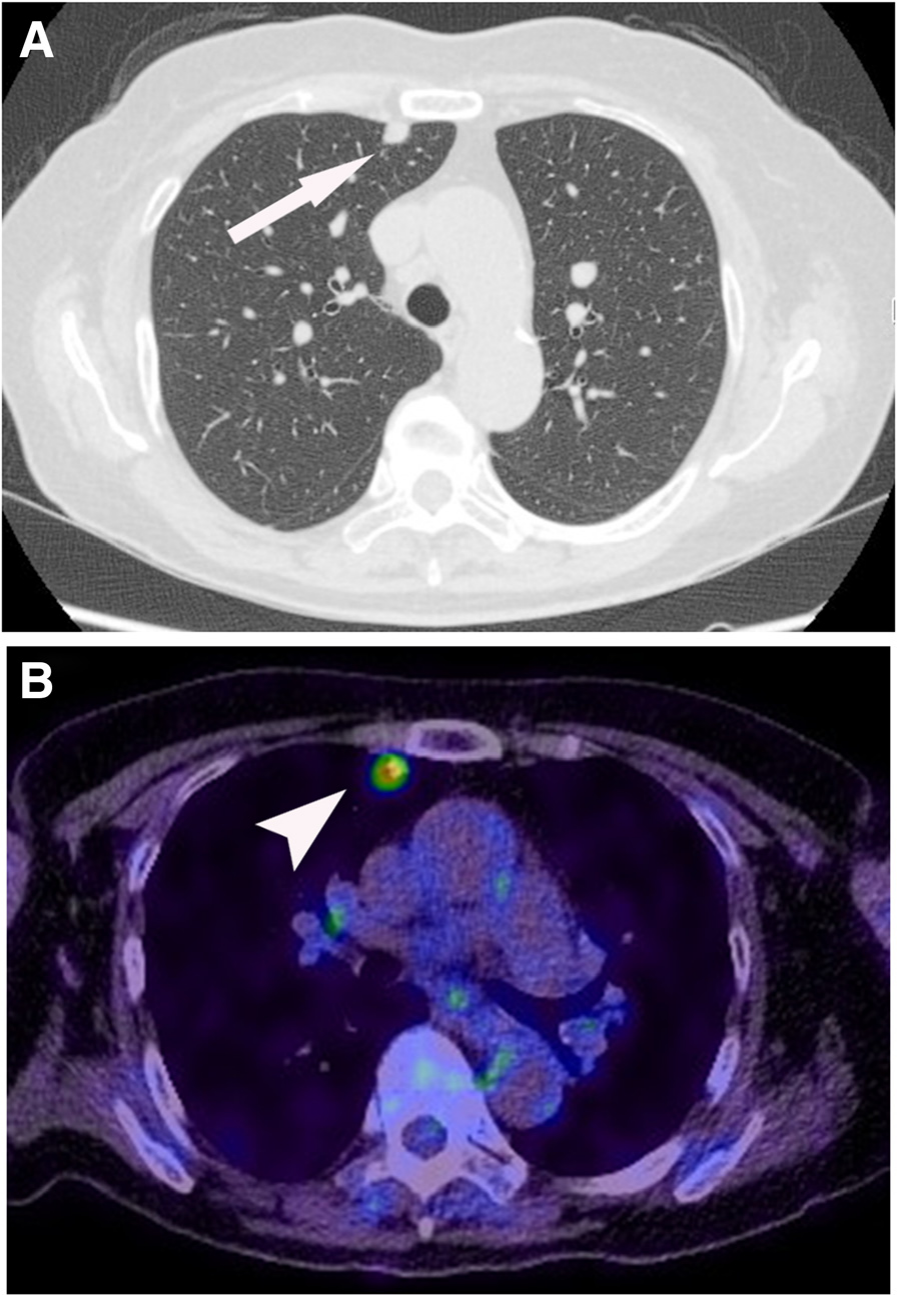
Computed tomography (CT) and positron emission tomography of the tumors. (A) Chest CT showed a 1.1 cm nodule in the anterior segment of the right upper lobe (arrow). (B) PET-CT showed fluorodeoxyglucose accumulation with a Standardized uptake value (SUV) of 3.7 (arrowhead).
